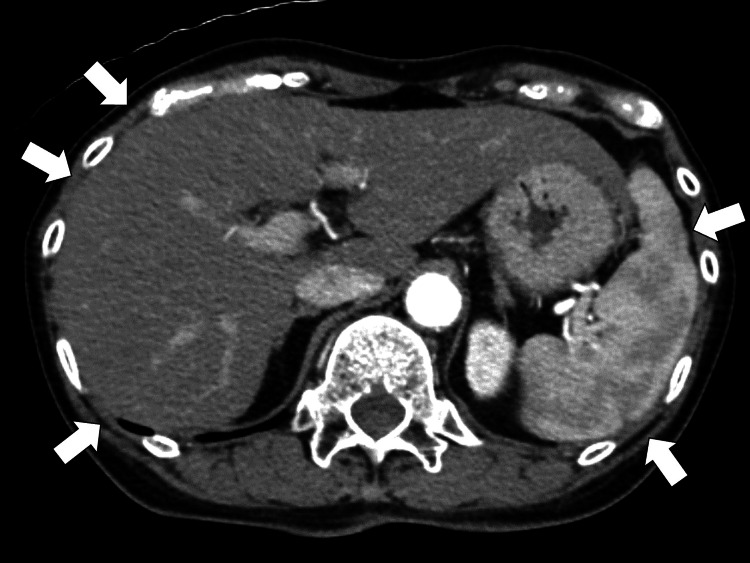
Contrast-enhanced computed tomography scans from the chest to the pelvis show hepatosplenomegaly without lymphadenopathy (white arrows).
